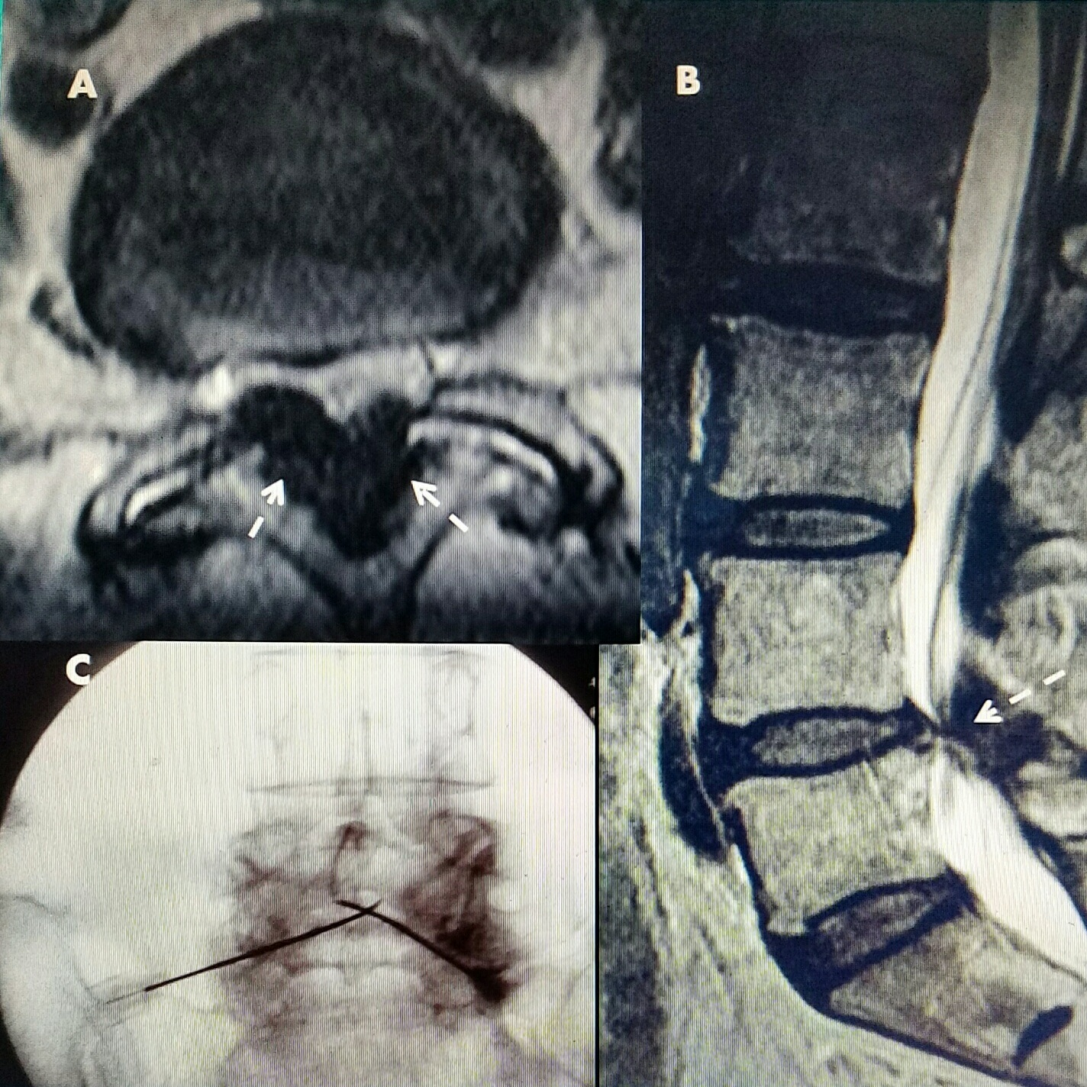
L4-5 stenosis radiofrequency electrode placement A: Axial CT showing marked ligamentous hypertrophy, (white arrows) which shows up hypointense and darker on T2 MRI. B: Sagittal T2 MRI with posterior compression from ligamentous stenosis at L4-5. C: Two RF electrodes placed percutaneously from inferior-laterally to superior-medially in posterior canal within ligamentum flavum. CT: Computed tomography; MRI: Magnetic resonance imaging; RF: Radiofrequency.
